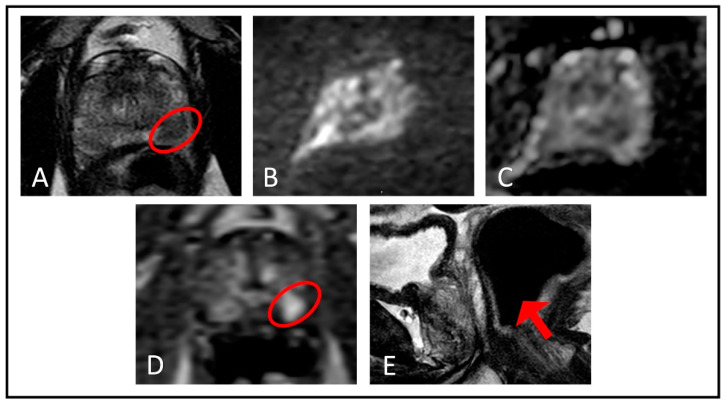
An example case from our own database demonstrating the added value of DCE MRI in the diagnosis of prostate cancer when DWI/ADC images are of insufficient quality, which may arise due to different reasons; in this case it was due to rectal gas-related distortion. (A) Axial T2-weighted imaging shows a hypointense lesion (red ellipse) in the left apical–mid peripheral zone. Both the (B) DWI and (C) ADC images are distorted due to rectal gas-related artifacts and unable to provide information regarding the hypointense lesion located in the left apical–mid peripheral zone. (D) Lesion demonstrates early focal enhancement (red ellipse) in dynamic contrast-enhanced imaging. (E) Rectal gas causing undiagnostic images in DWI and ADC sequences is depicted in the sagittal T2-weighted MRI (red arrow).
